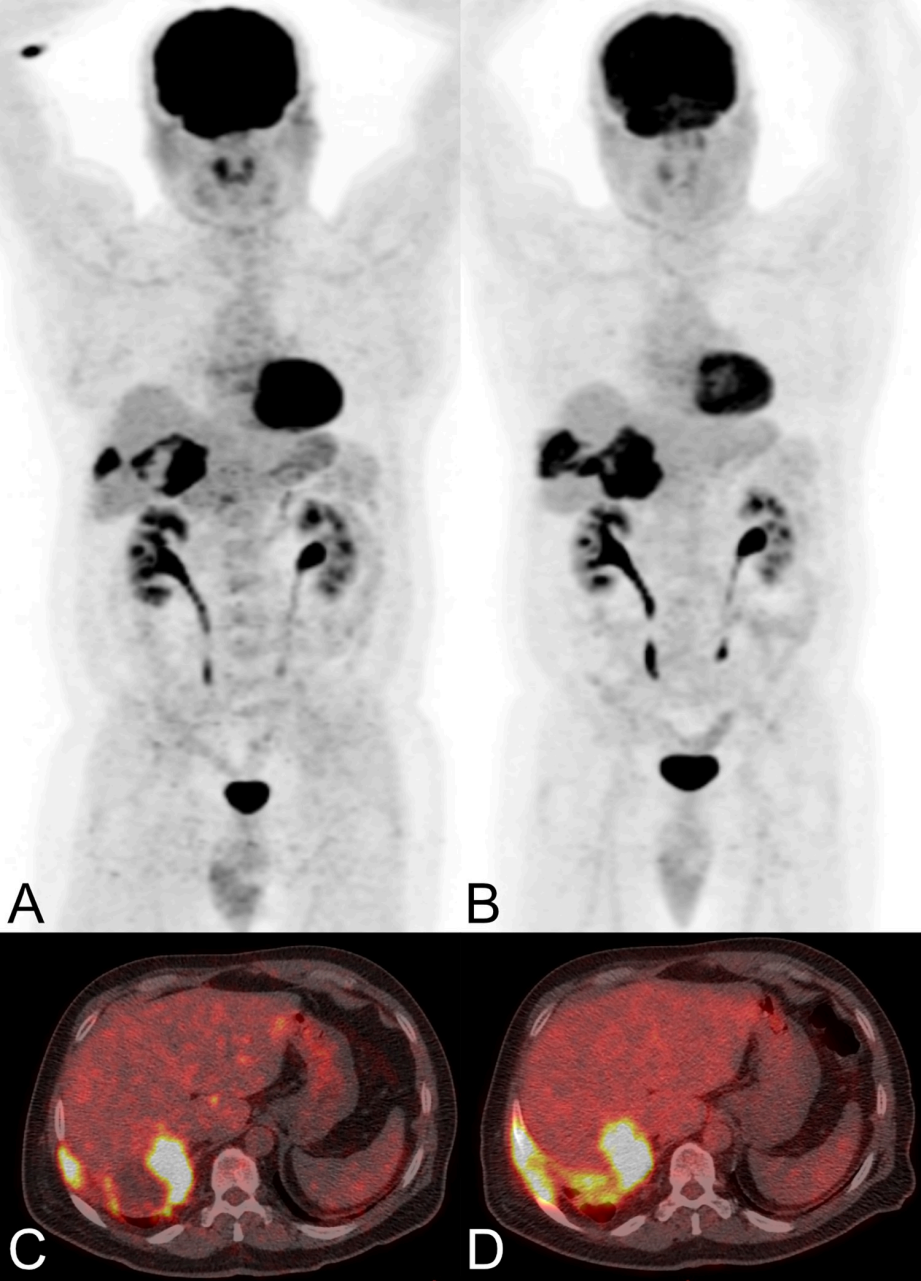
Two PET/CT scans performed in a male patient with a hepatic manifestation of alveolar echinococcosis involving the chest wall. The patient (patient 09 in Table 2) was 55 years old in 2008 (maximum intensity reconstructions of PET (A) and fused PET/CT images (C)), and 58 years old in 2011 (B and D) and was treated with mebendazole from 2001 to 2011. The follow-up PET/CT 2011 (B and D) documented progression of disease with increasing involvement of the chest wall as compared to the PET/CT from 2008 (A and C) (EgHF AU increased from 34 to 35, SUVratio liver was continuously high at 4.6), and therapy was subsequently changed to albendazole. The patient died in 2017 of unknown cause.
